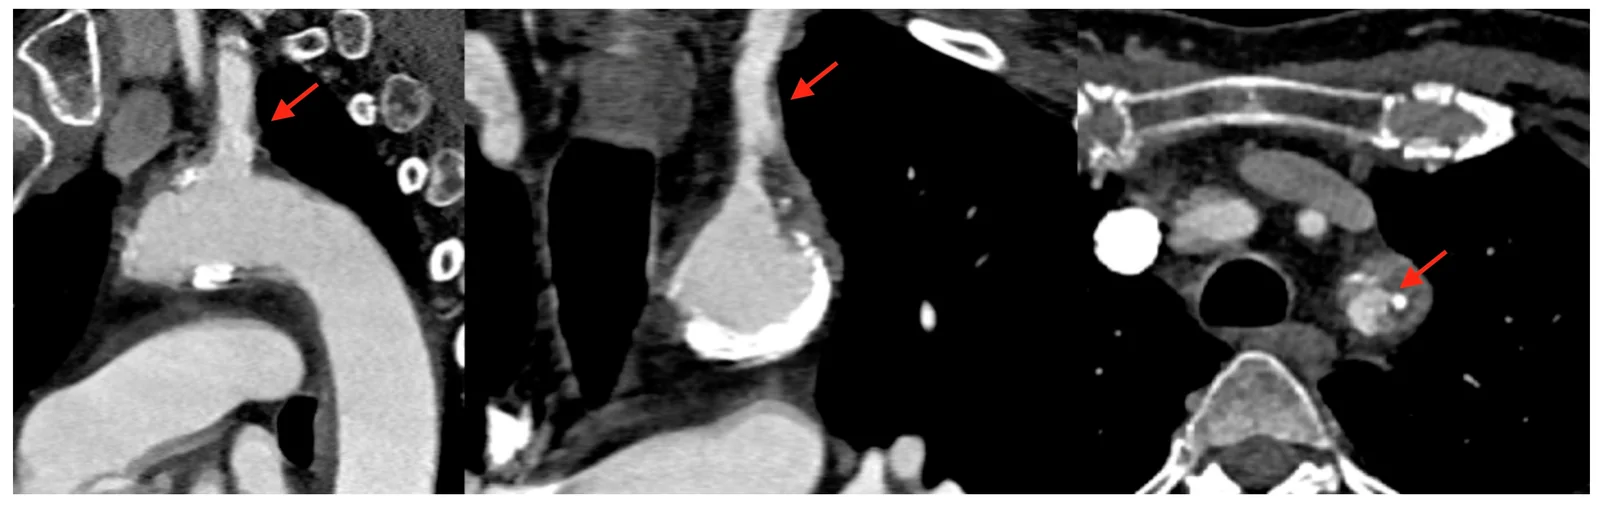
Computed tomography angiography after the procedure. Representative multiplanar reconstruction showing substantial resolution of stenosis within the left subclavian artery (arrow).
